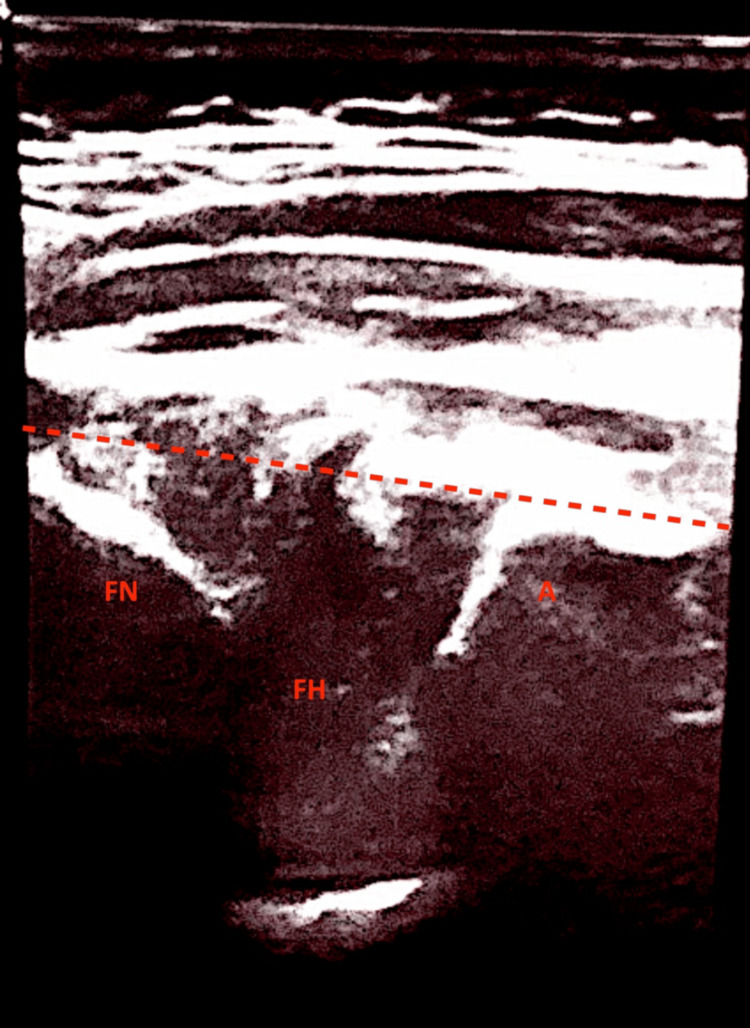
Anterior hip ultrasound using the van Douveren method, demonstrating disruption of the modified Shenton line, indicating hip dislocation A: acetabulum; FH: femoral head; FN: femoral neck
